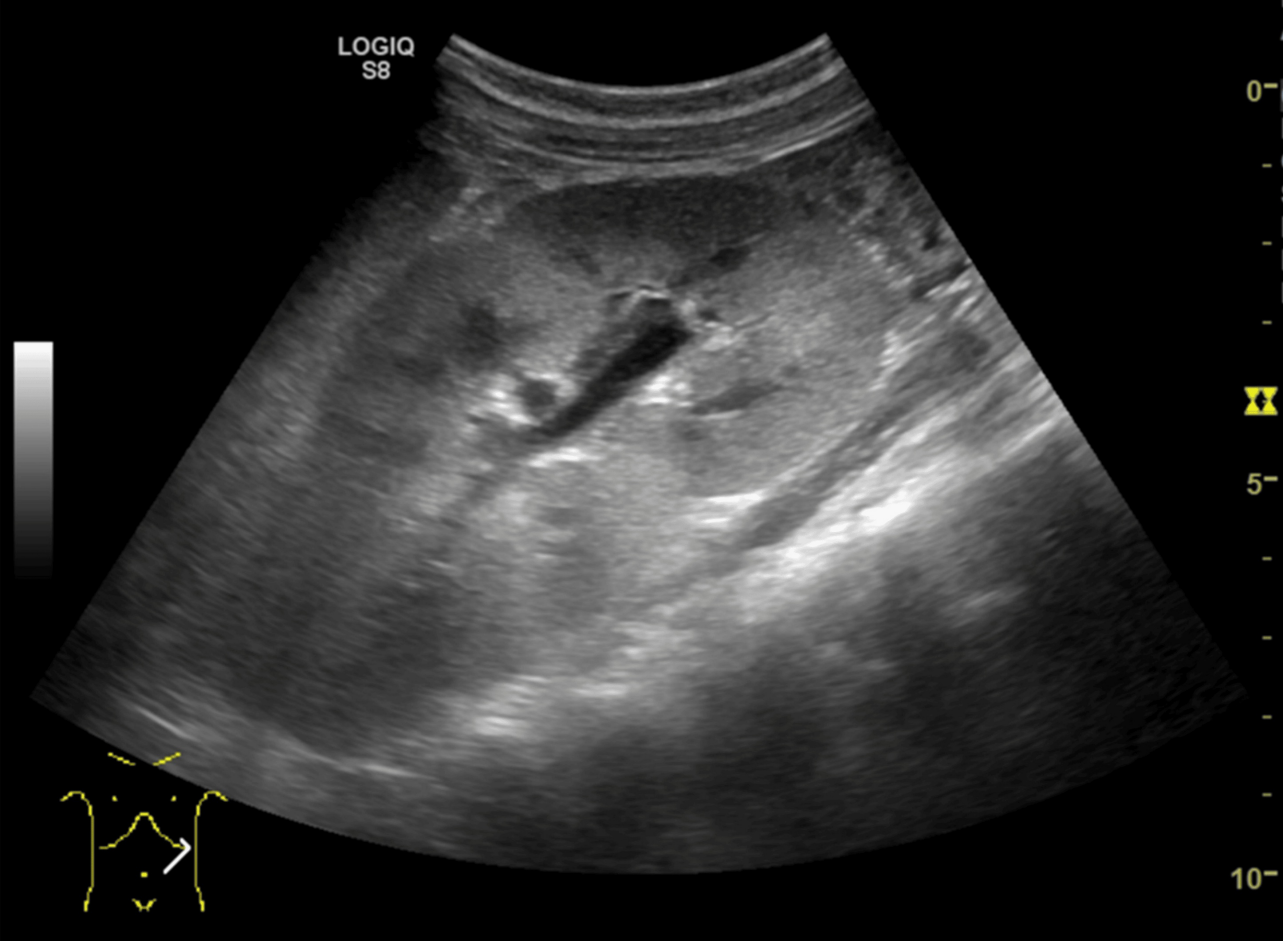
Ultrasound image of the left kidney showing increased echogenicity of the renal parenchyma and renal swelling.
